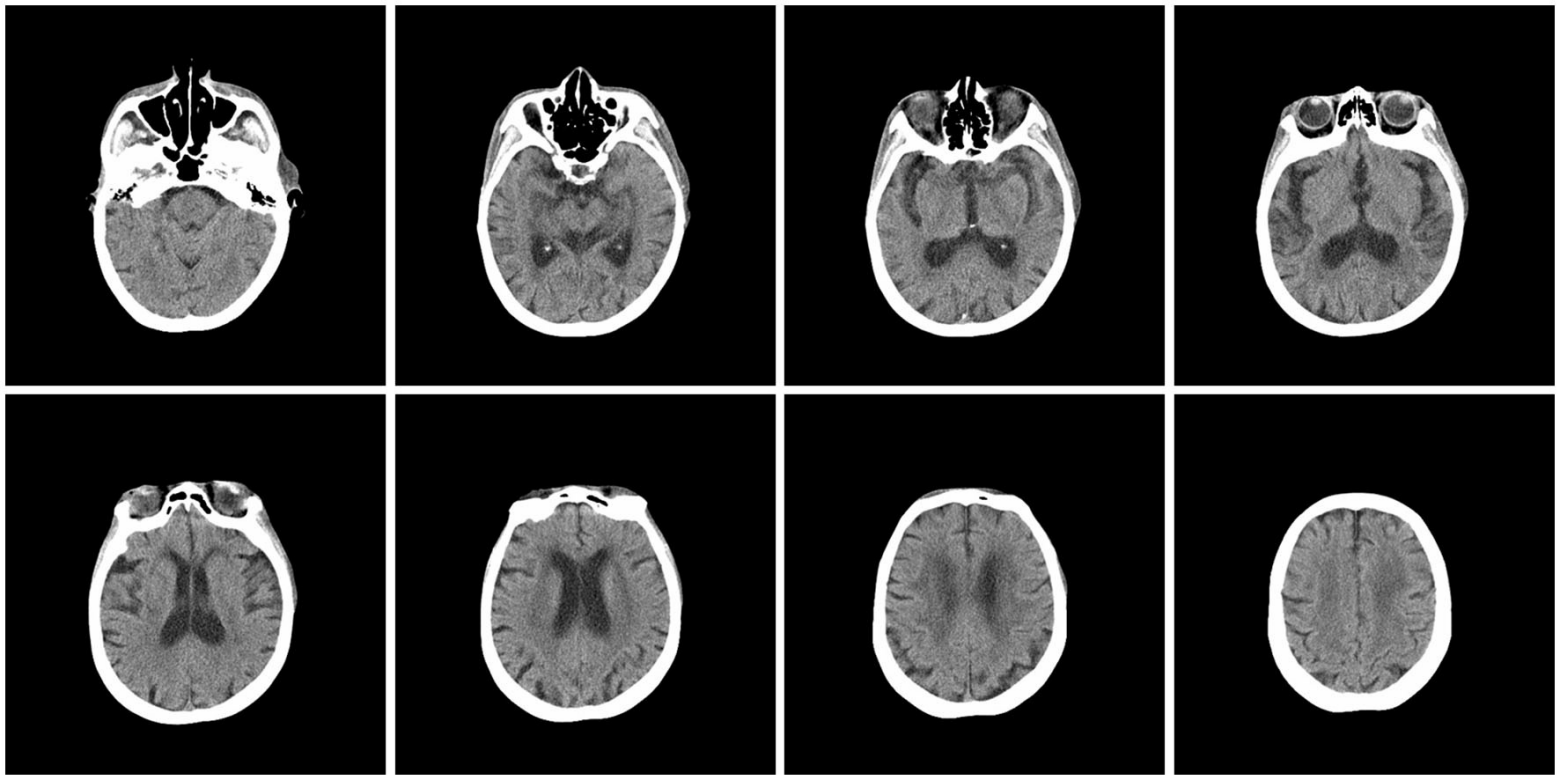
Head CT basically ruled out intracranial hemorrhage, head injury and strategic infarction.
